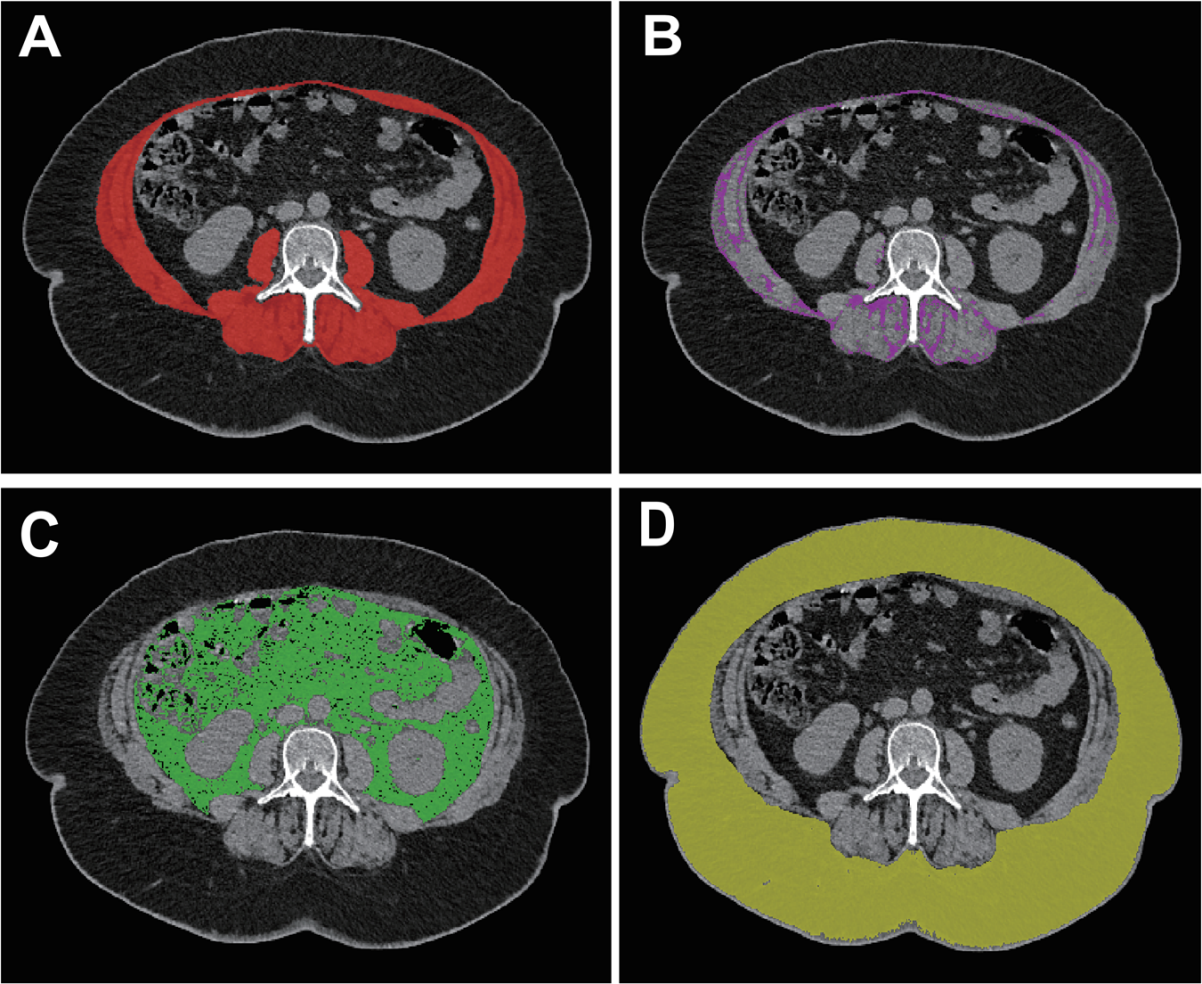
Computed tomography image (L3) shows the different tissue segmentations after thresholding in a patient with liver metastasis before ^90^Y-radioembolization. The color-coded areas represent the skeletal muscle area (A), intermuscular adipose tissue area (B), visceral fat area (C), and subcutaneous fat area (D)
